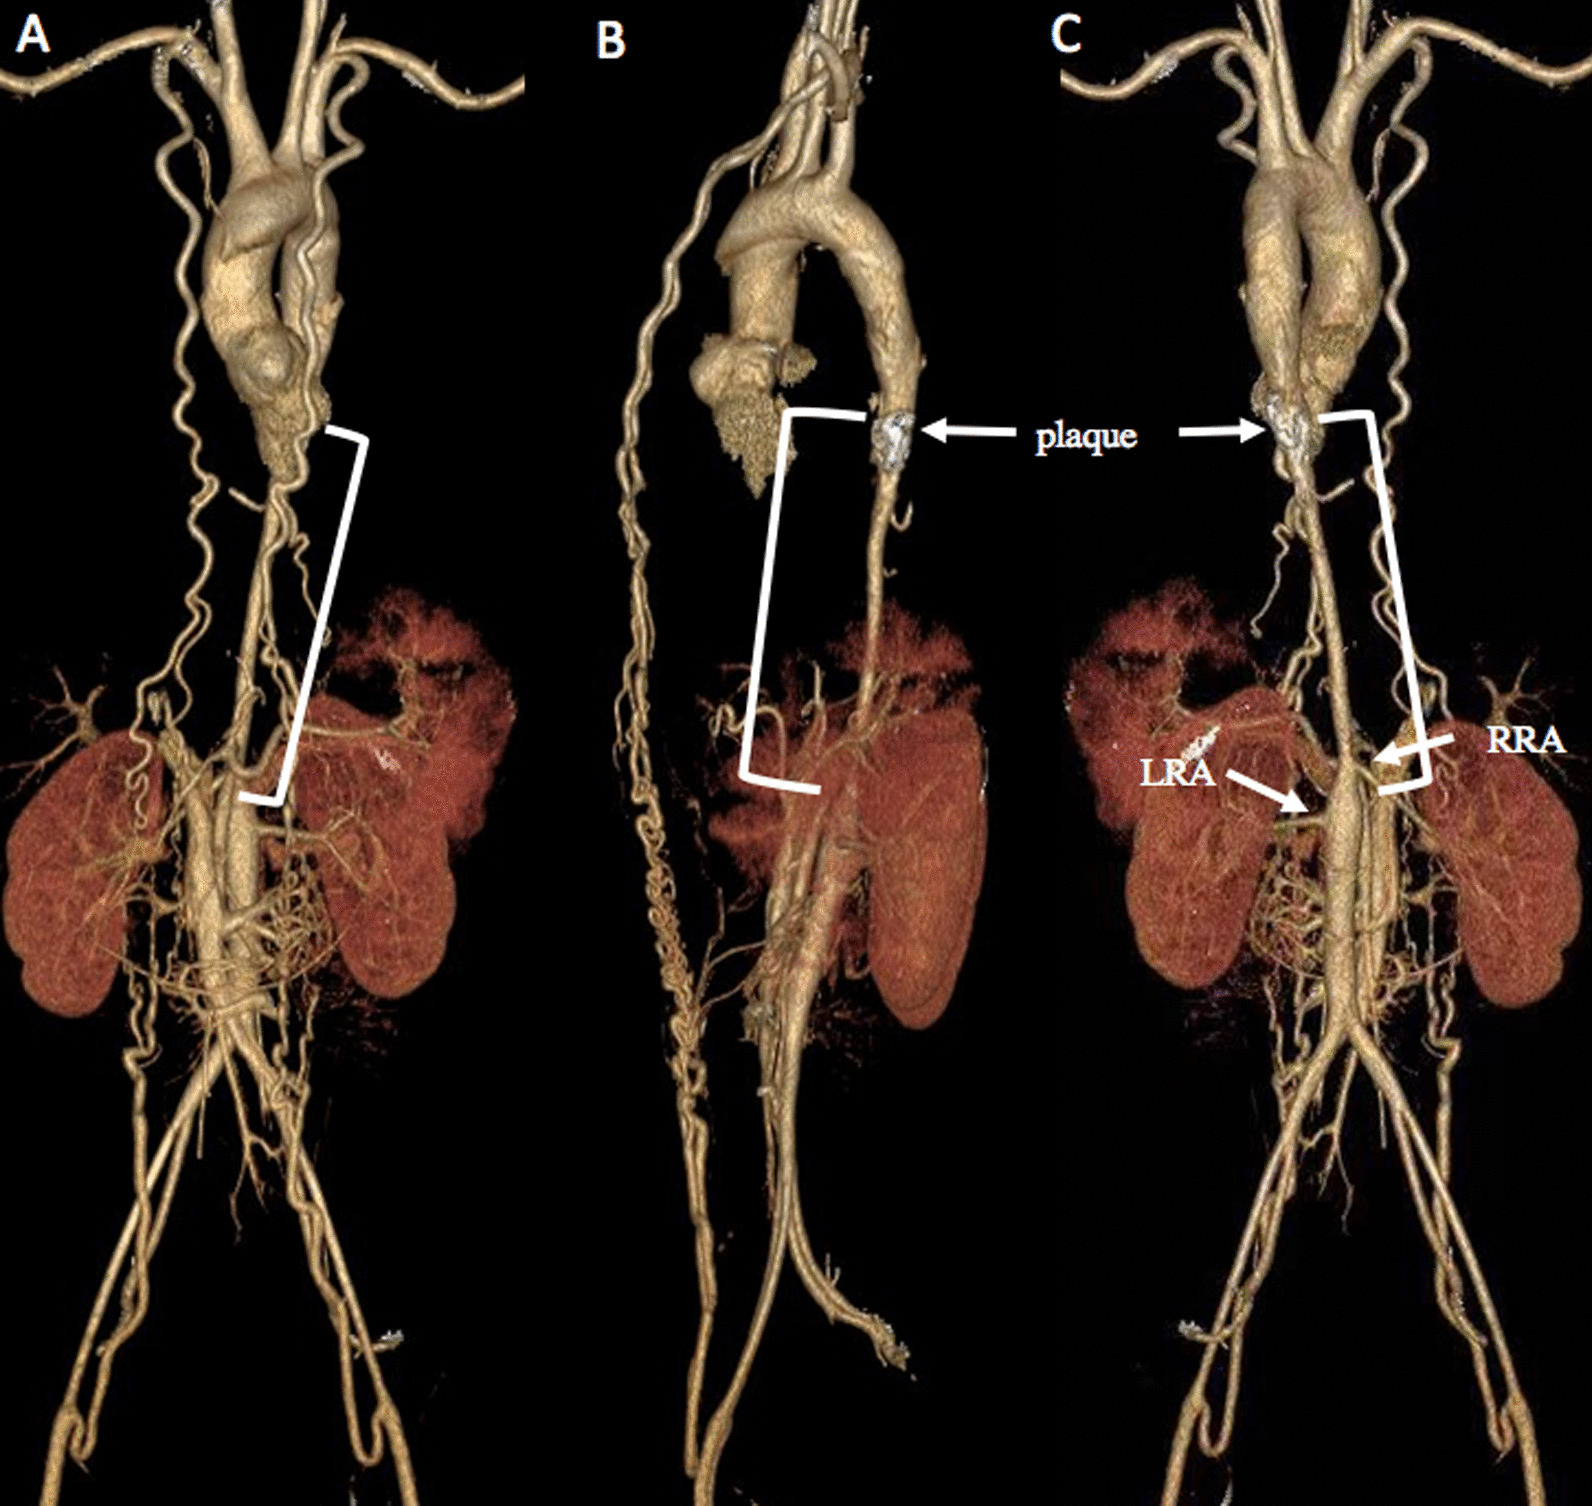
CTA imaging demonstrated a long-segment narrowing (bracket) in the descending aorta at the level of T8–L2 vertebra, with a calcified plaque in the stenotic aorta, RRA involvement and a rich network of collateral vessels between the pre-and post-stenotic region. A Frontal view; B Lateral view; C Posterior view. *LRA* Left renal artery, *RRA* Right renal artery
